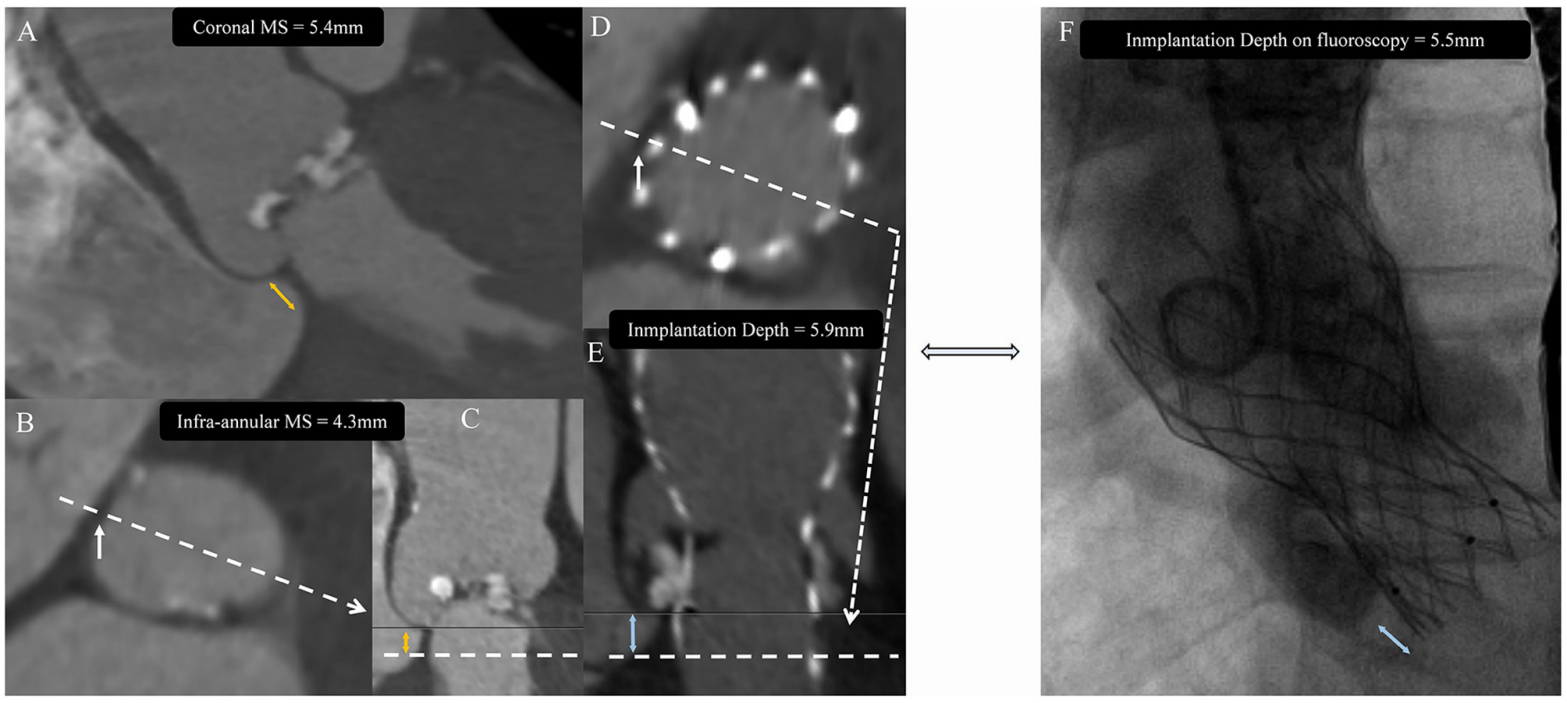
Image analysis protocol. The membranous septum (MS) was measured on coronal view (A) as coronal MS or on stretched vessel view (C) as infra-annular MS at the tricuspid insertion point (B). Device's implantation depth (ID) was measured on post-operative CT (D,E) and was compared with the measurement on post-releasing fluoroscopy (F). Yellow double arrow indicates membranous septum length (MS). White arrow indicates tricuspid insertion point. Blue double arrow indicates prosthesis implantation depth (ID).
